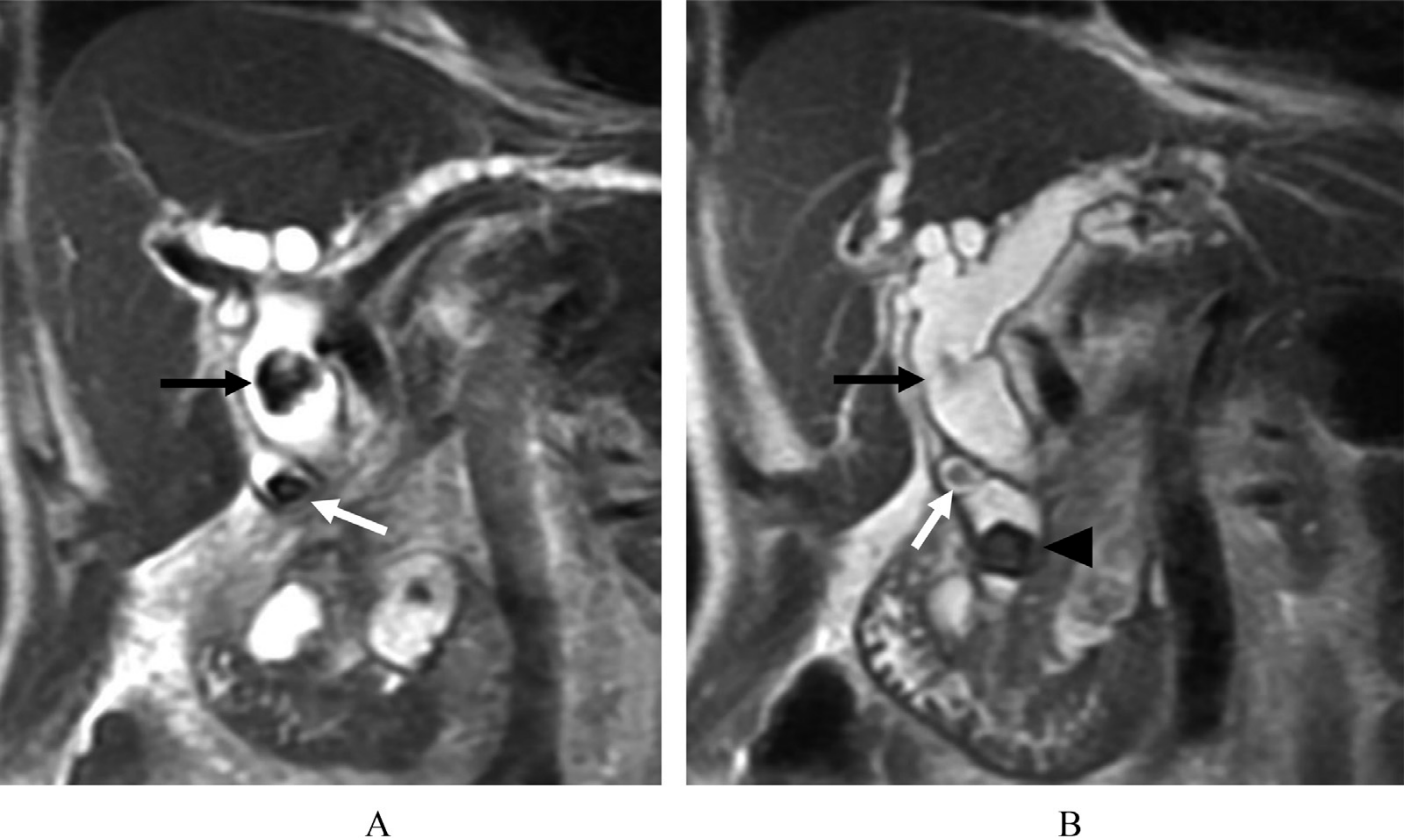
(A) Coronal image of T2-weighted MRI shows common hepatic duct stone (black arrow) and cystic duct stone (white arrow). (B) Another coronal image of T2-weighted MRI shows cystic duct stone (white arrow) and common bile duct stone (arrowhead).
